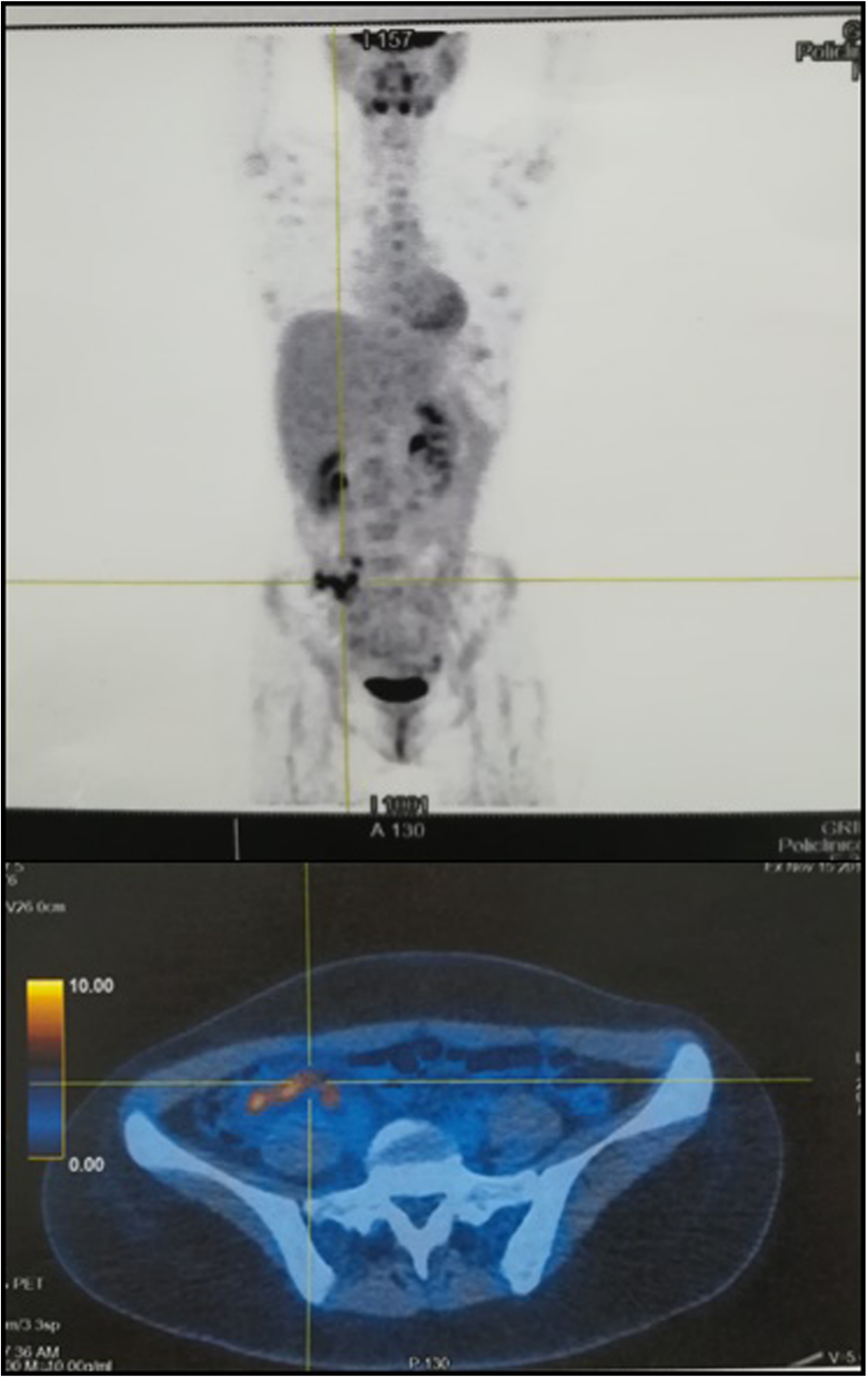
Second CT/PET Total Body. The second CT/PET shows ^18^F-DFG accumulation only in the descending colon
